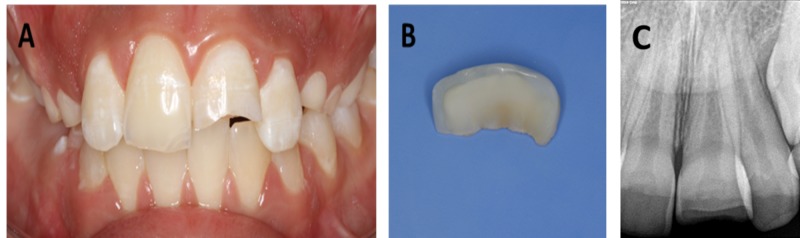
Pre-operative images. (A) Fractured maxillary central incisor. (B) Fractured fragment of the maxillary central incisor. (C) Peri-apical radiograph of the broken maxillary central incisor.
